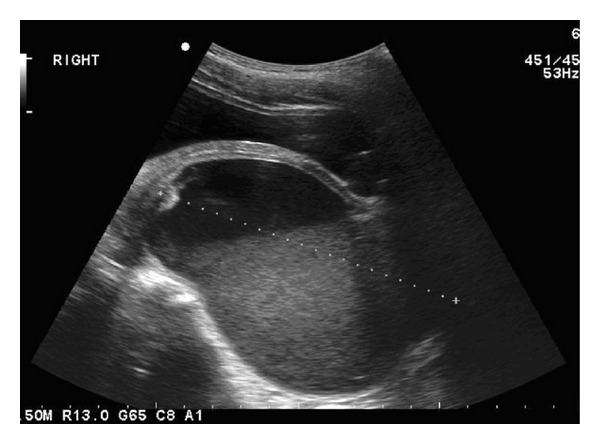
Ultrasound scan showing fluid-filled uterus.
